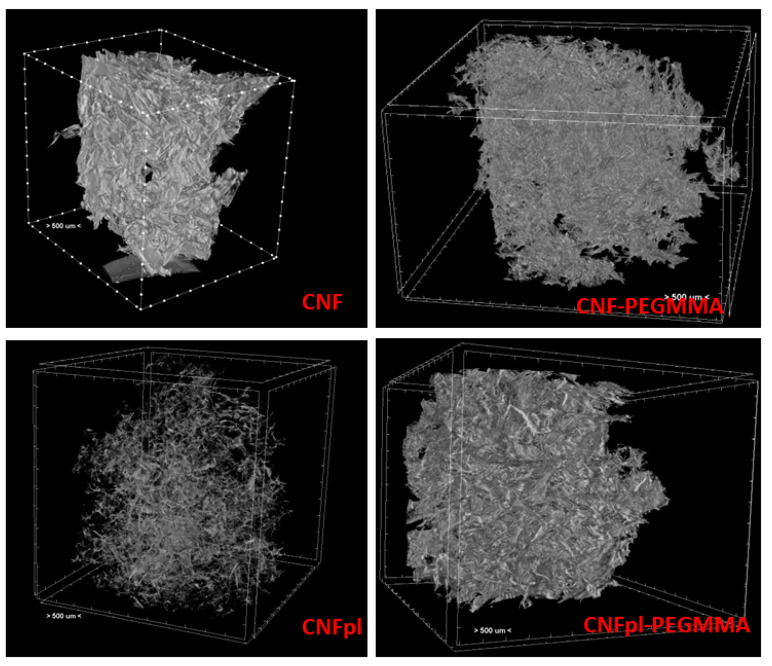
μ-CT images of ungrafted and PEGMMA-grafted CNF and CNFpl sponges.
